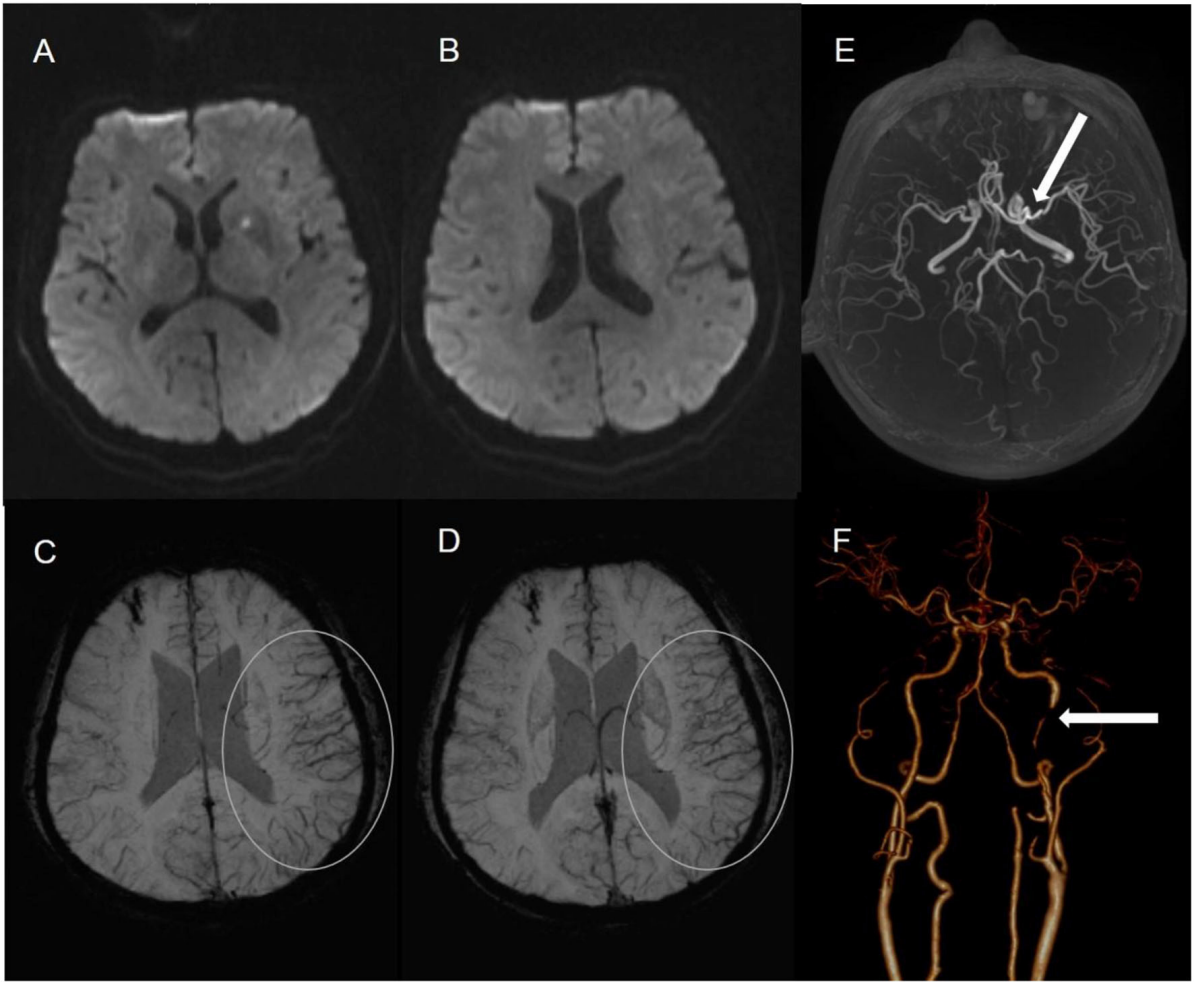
A 61-male patient with left LI (A,B). SWI indicates APCV in the left hemisphere [(C,D) white circle]. CTA showed left internal carotid artery occlusion [(F) white arrow]. The status of cerebral arterial circle was graded as good with the presence of contrast filling from the M1 to M3 of MCA and anterior cerebral artery [(E) white arrow].
